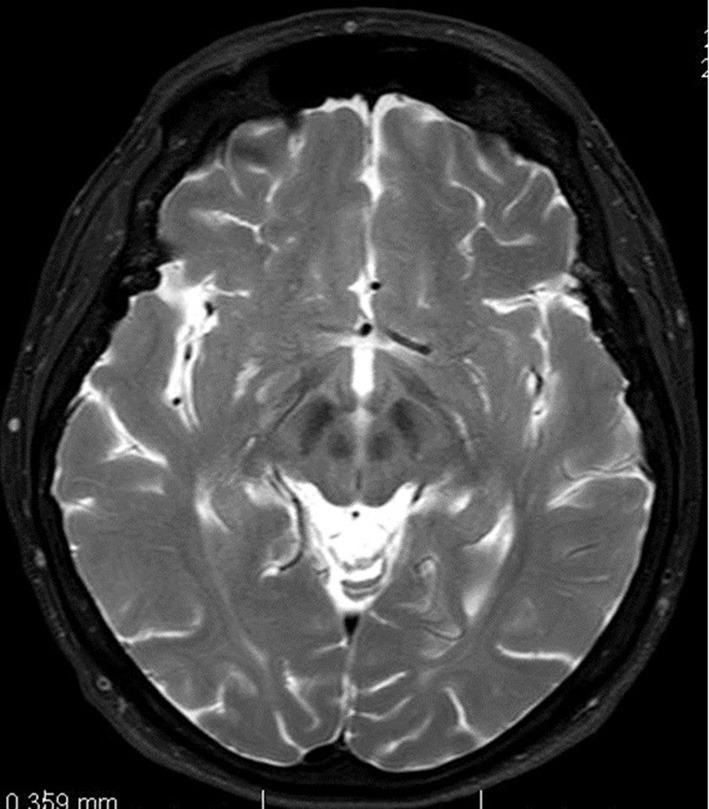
Magnetic resonance imaging of brain. T2-weighted axial scan. Changes in midbrain retrospectively evaluated as so-called face of giant panda sign.
